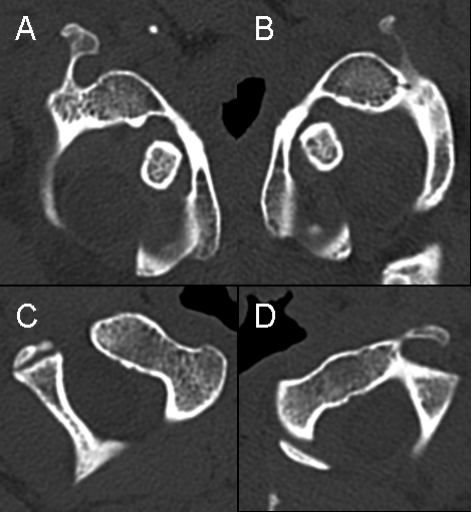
Rotatory computed tomography scan. Atlas (A + B) and axis (C + D) with the head rotated to the left (A + C) and to the right (B + D).
